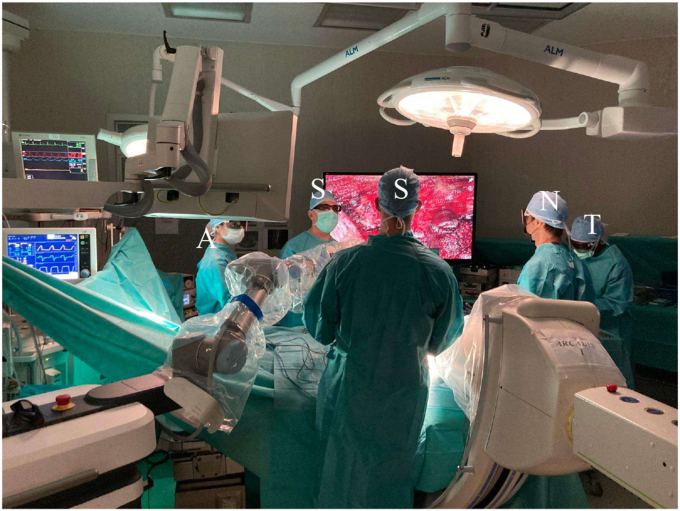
Operating room setup with the exoscope. Two surgeons (S) face each other, viewing the large monitors with their heads in a physiological posture. The anesthesiologist (A), scrub nurse (N), and X-ray technician (T), all wearing 3D glasses, actively observe the monitors, highlighting the coordinated participation of the entire surgical team throughout the procedure.
